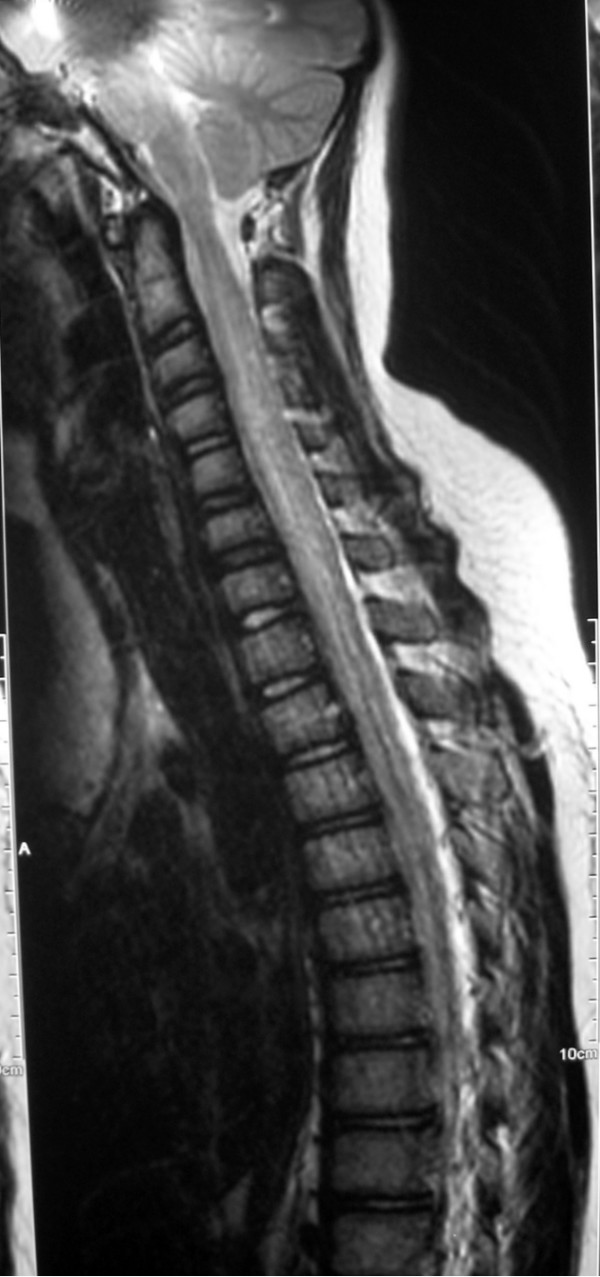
Sagittal T2-weighted magnetic resonance image of spine at admission. There is longitudinal hyperintense signal involving the central cord from C1 downwards.
